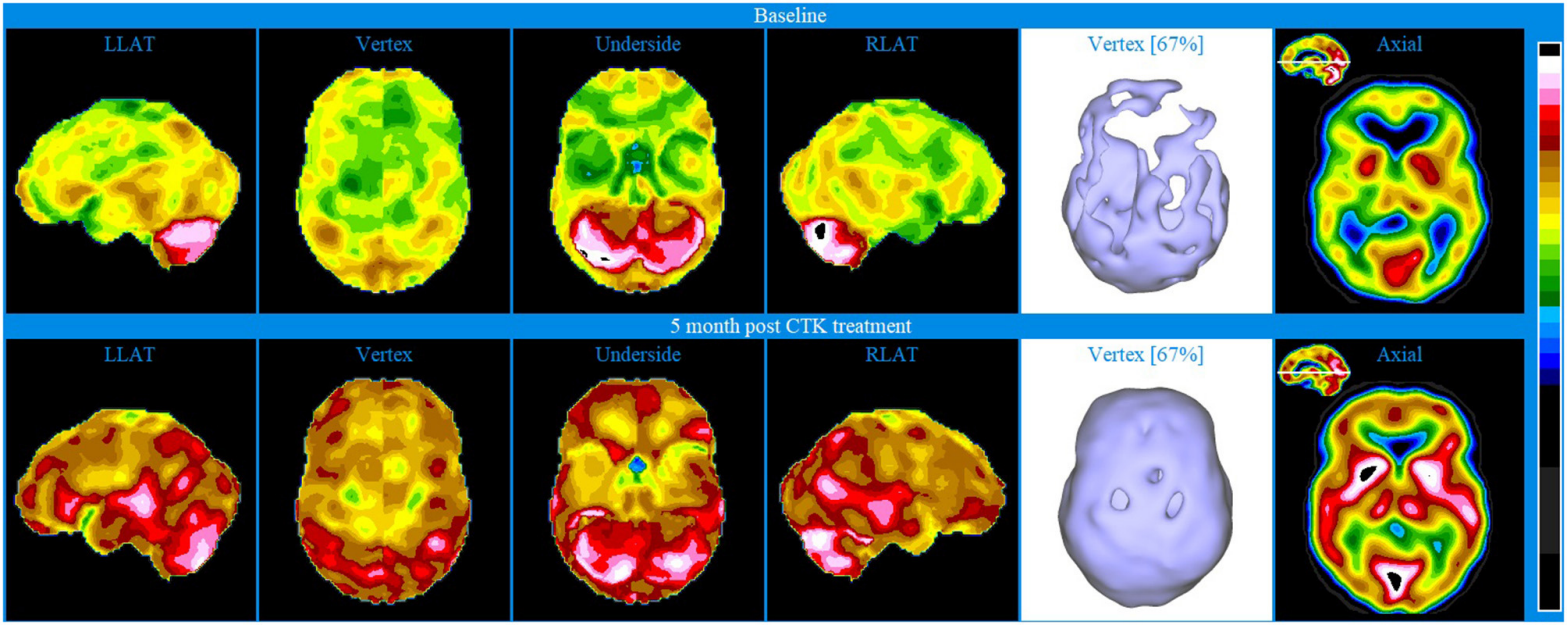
Brain SPECT images from Patient I. Top line images relate to brain SPECT images at baseline. Bottom line images are taken following 5 months of CTK treatment. The six images on each row (from left to right) include four stereotactic surface projections of the left lateral (LLAT), vertex, underside and right lateral (RLAT), one iso-surface image of the vertex and one axial slice. Color-coded intensity indicates hyperfunctioning areas (blue hues) and hypofunctioning areas (white and black surrounded by white). Images are presented as described in the Methods.
